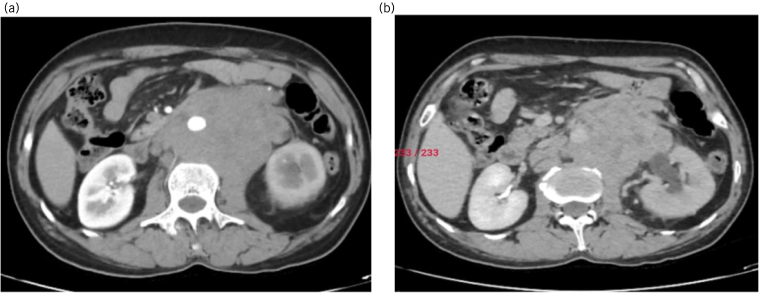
Contrast-enhanced computed tomography abdomen and pelvis scan showing a retroperitoneal lymph nodal mass (a) encasing the aorta and (b) the effect of pressure on the left ureter resulting in hydroureteronephrosis.
